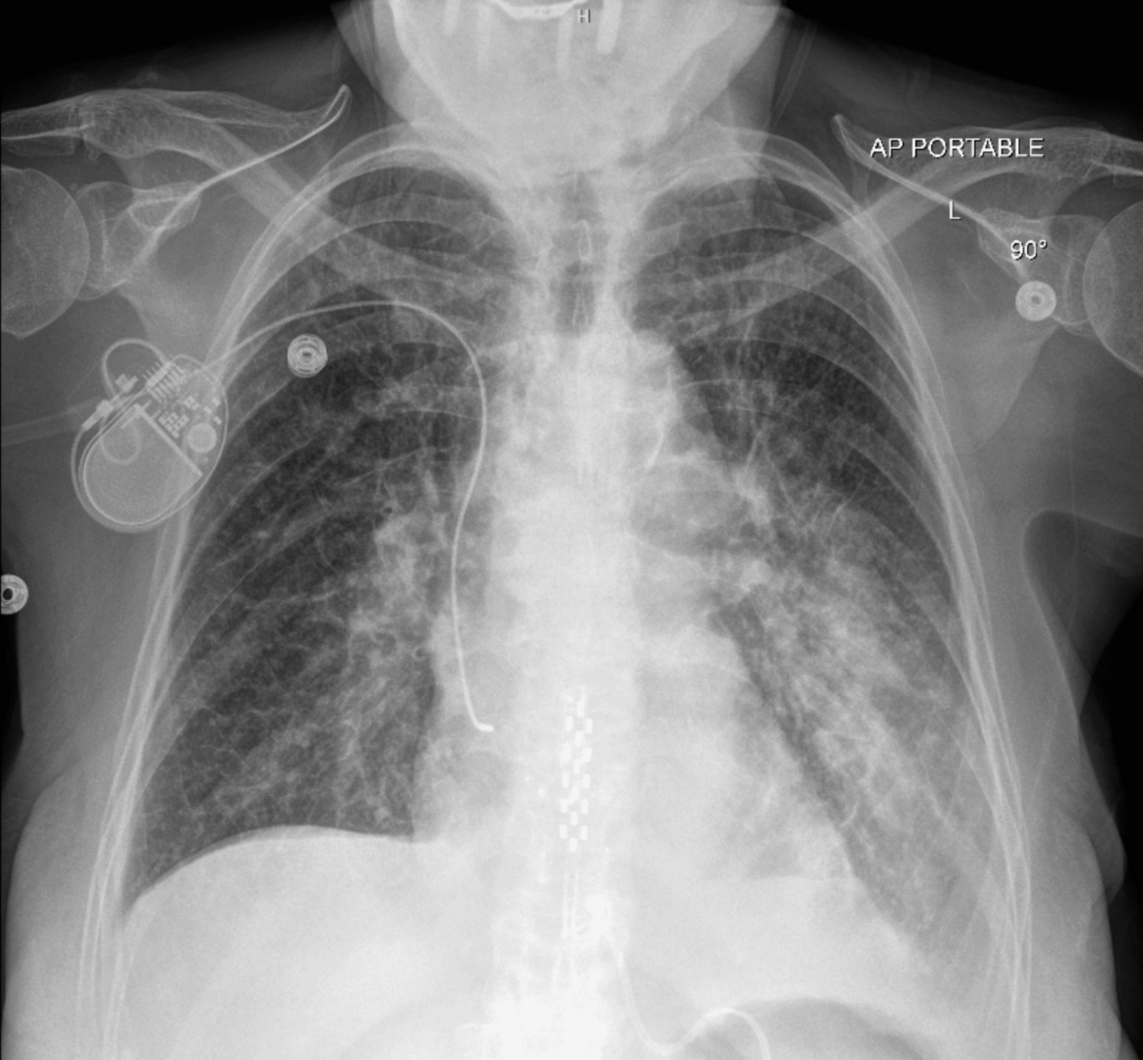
Chest X-ray demonstrating diffuse interstitial markings consistent with pulmonary edema.
